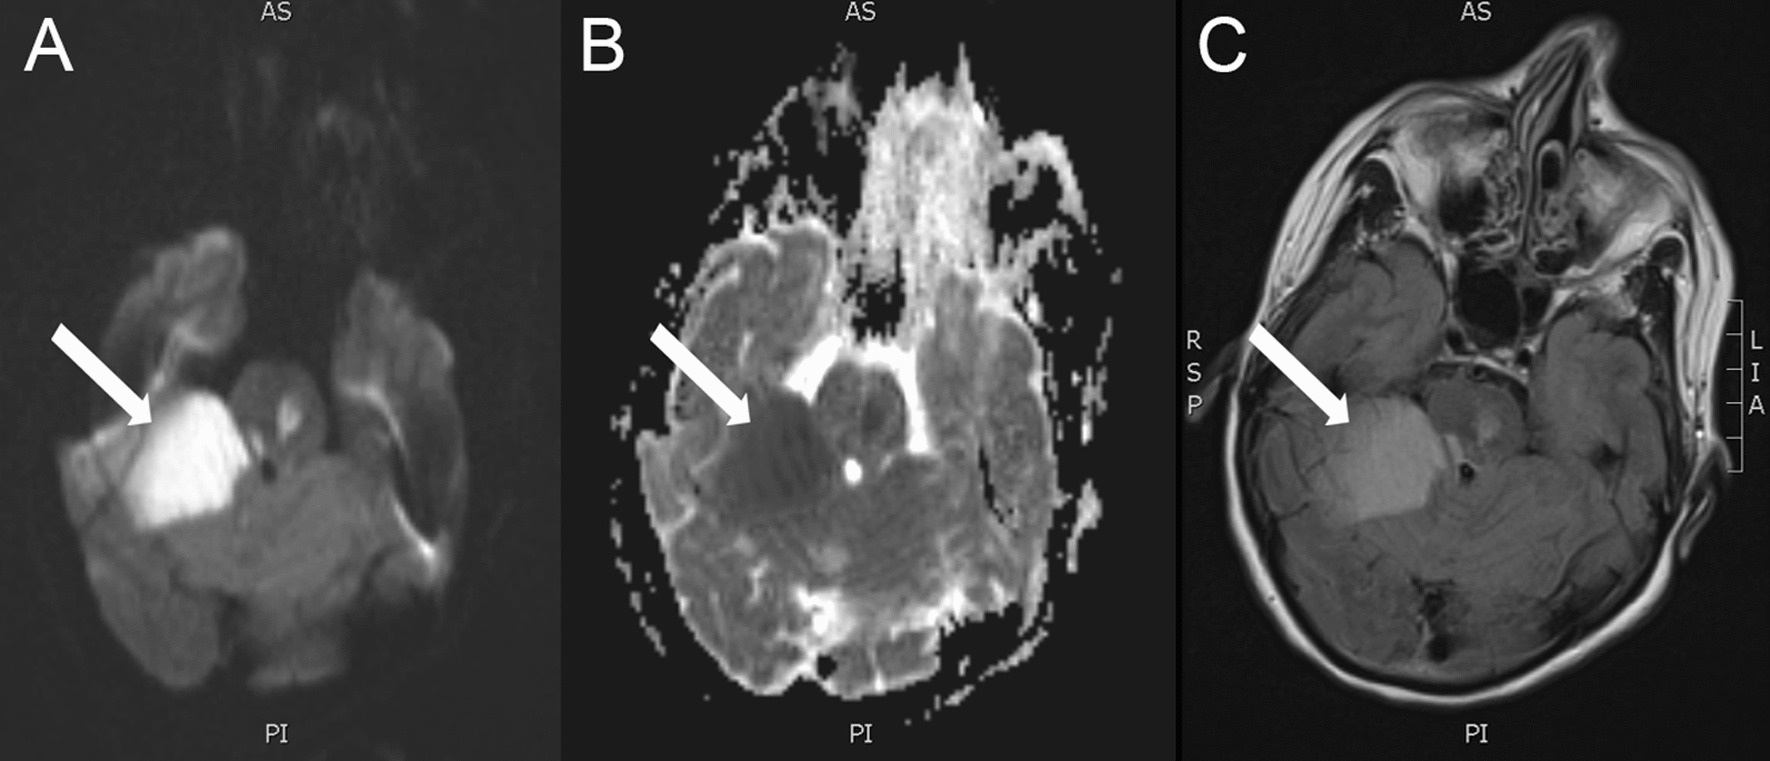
MR Brain with DWI (A), ADC (B), and FLAIR (C) sequences. The white arrows demonstrate a large territory of restricted diffusion in the right cerebellar hemisphere, with an additional focus in the left pons
